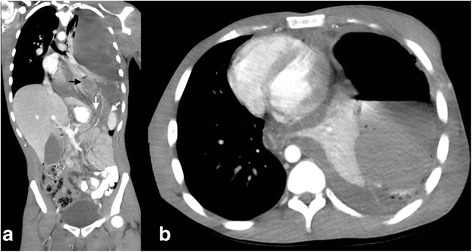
Computed tomography. a Coronal view demonstrating hernia of diaphragm containing large bowel and a complex hydropneumothorax of the left thoracic cavity consistent with perforation of the herniated bowel causing faecopneumothorax. Black arrow points to a loop of large bowel within the thoracic cavity, and white arrows point to the diaphragmatic hernia defect. b Axial view demonstrating collapse of the left lung due to the complex hydropneumothorax and contralateral mediastinal shift
